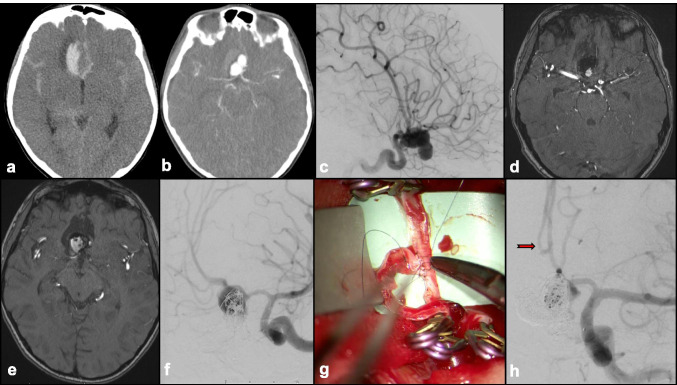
A 10-year-old patient presented with coma producing aneurysmal SAH. Preintubation, his best motor score was flexing. a CT head showed diffused SAH, with associated frontal hematoma. b CT angiogram confirmed an underlying complex multilobulated anterior communicating artery (ACommA) aneurysm. c Cerebral angiogram (R ICA injection) showed the multilobulated ACommA, and right A2 arising from the neck of the aneurysm. He underwent coil occlusion of the ruptured ACommA, securing the ruptured fundal component. d MR angiogram, small neck remnant of coiled aneurysm was evident to protect the right A2 branch. After a period of ICU stay and rehabilitation, he made good recovery. e MR angiogram at 6 months follow-up showed significant aneurysm recurrence. f Cerebral angiogram (left ICA) injection showed ACommA aneurysm enlargement. After multidisciplinary discussion, we proceeded to perform right pericallosal to left pericallosal in situ (IC-IC) bypass, prior to further coil occlusion of the aneurysm, sacrificing the right A2. g Intraoperative view of pericallosal-pericallosal in situ bypass (IC-IC), end to side technique using interrupted 9/0 suture. h Angiogram after further coil occlusion of the recurrent ACommA aneurysm, and left A2 supplying both pericallosal branches (red arrow)
